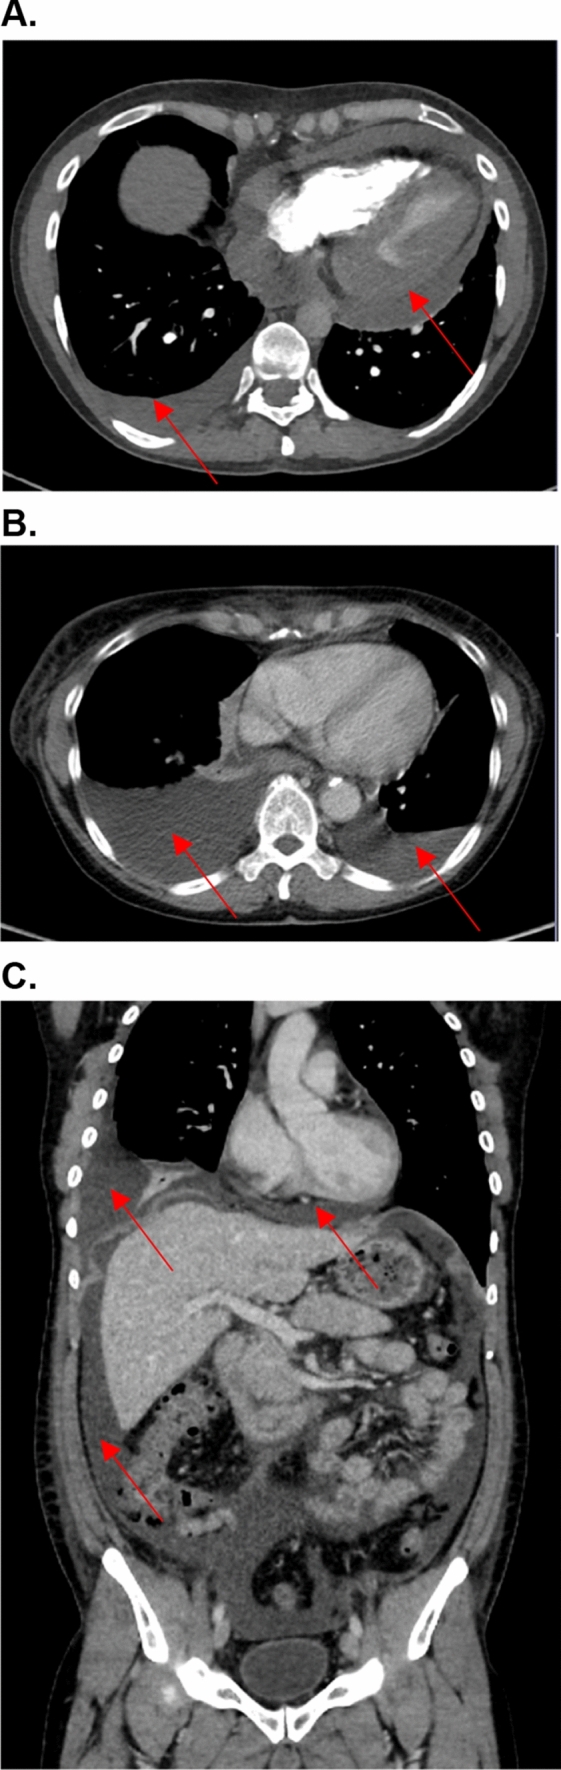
A Case 1. CT angiography of the arteria pulmonalis. Pulmonary arterial contrast phase to rule out pulmonary embolism. Circular pericardial effusion with rim up to about 2 cm and low density enhancement up to about 20–30 HU (Hounsfield units). Furthermore, serous pleural effusion on the right of approximately 2 cm. B Case 3. PET/CT with 232 MBq F-18 FDG, venous phase. Serous pleural effusions (approximately 15 Hounsfield units). Right > left up to 6.2 cm. C Case 4. Marked four-quadrant ascites (approximately 5–10 Hounsfield units (HU)). Basal serous pericardial effusion approximately 1.5 cm (up to 10–15 HU) as well as serous pleural effusion on the right
